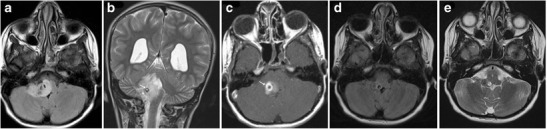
6 year old with headache, vomiting and fever: a Axial FLAIR image reveals hyperintense signal involving the medulla with extension into the adjacent cerebellar white matter. b and c Coronal T2-weighted image reveals small lesion with peripheral hypointensity at pontomedullary junction (*arrow*), the lesion shows peripheral enhancement on post contrast images. Possibility of CNS tuberculoma was considered. d–e Follow-up after 3 months on anti-tubercular treatment: Axial FLAIR and T2-weighted images show significant reduction in the hyperintensity involving the medulla with residual lesions. Clinically the child showed significant improvement
